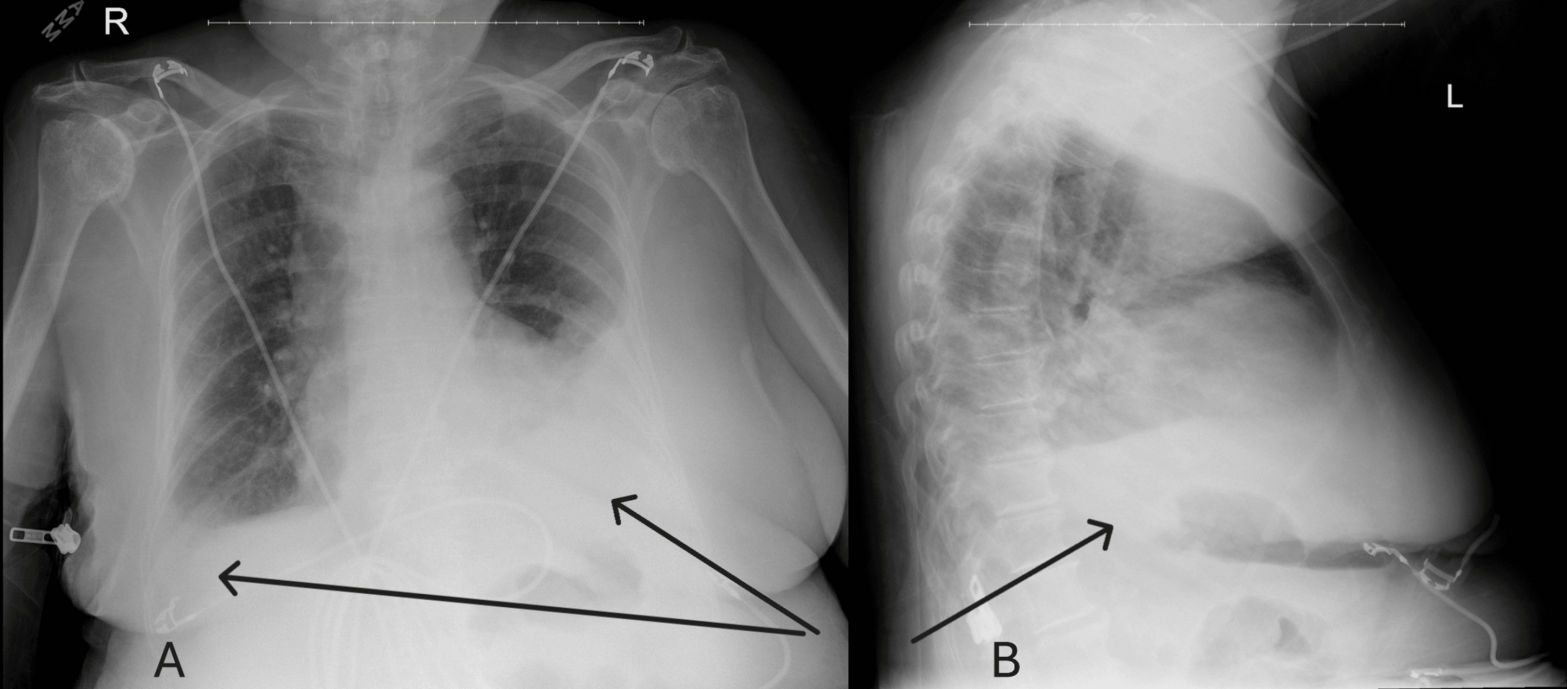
A: Anterior-Posterior Chest X-Ray. B: Lateral Chest X-Ray. Note the bilateral pleural effusions.
